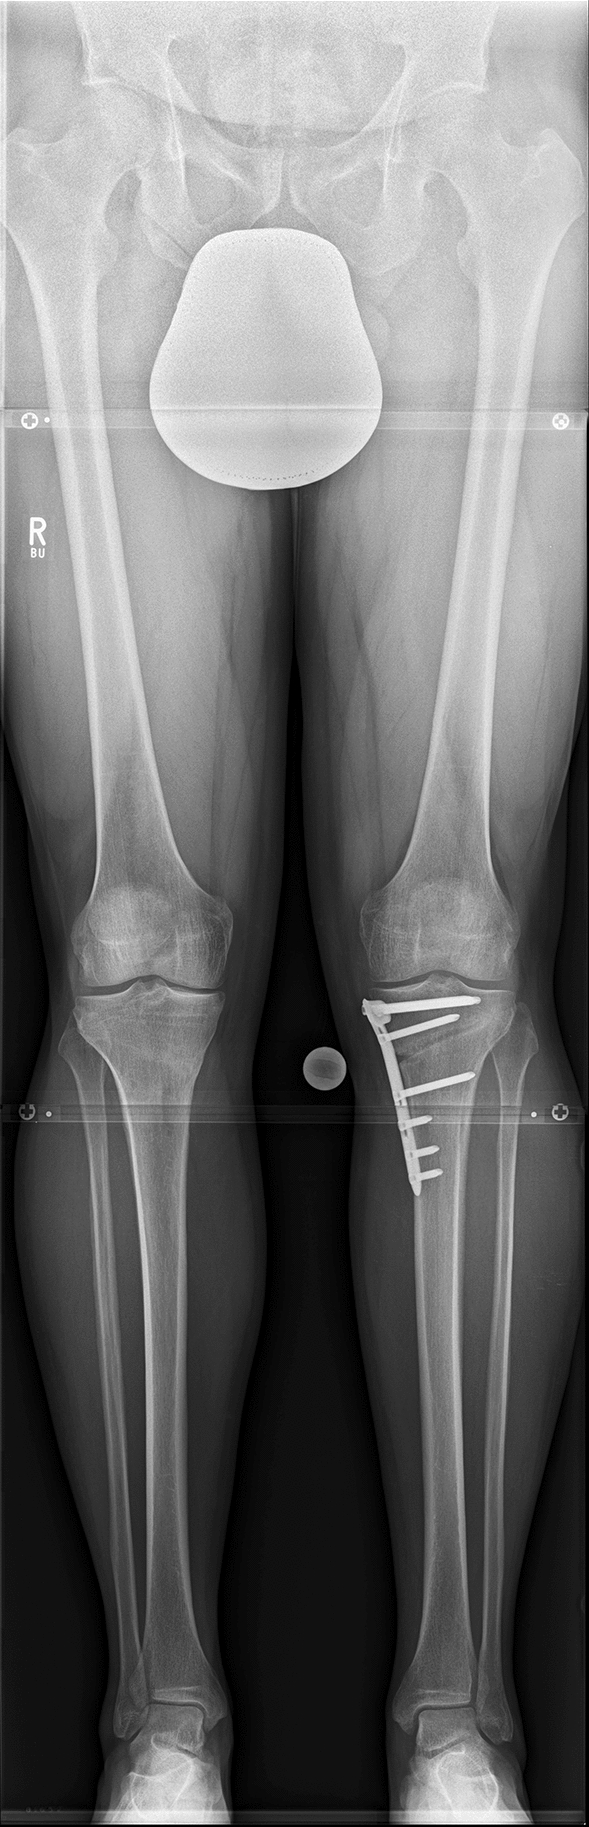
Anteroposterior long-leg weight-bearing radiograph after high tibial osteotomy for valgisation of genu varum
